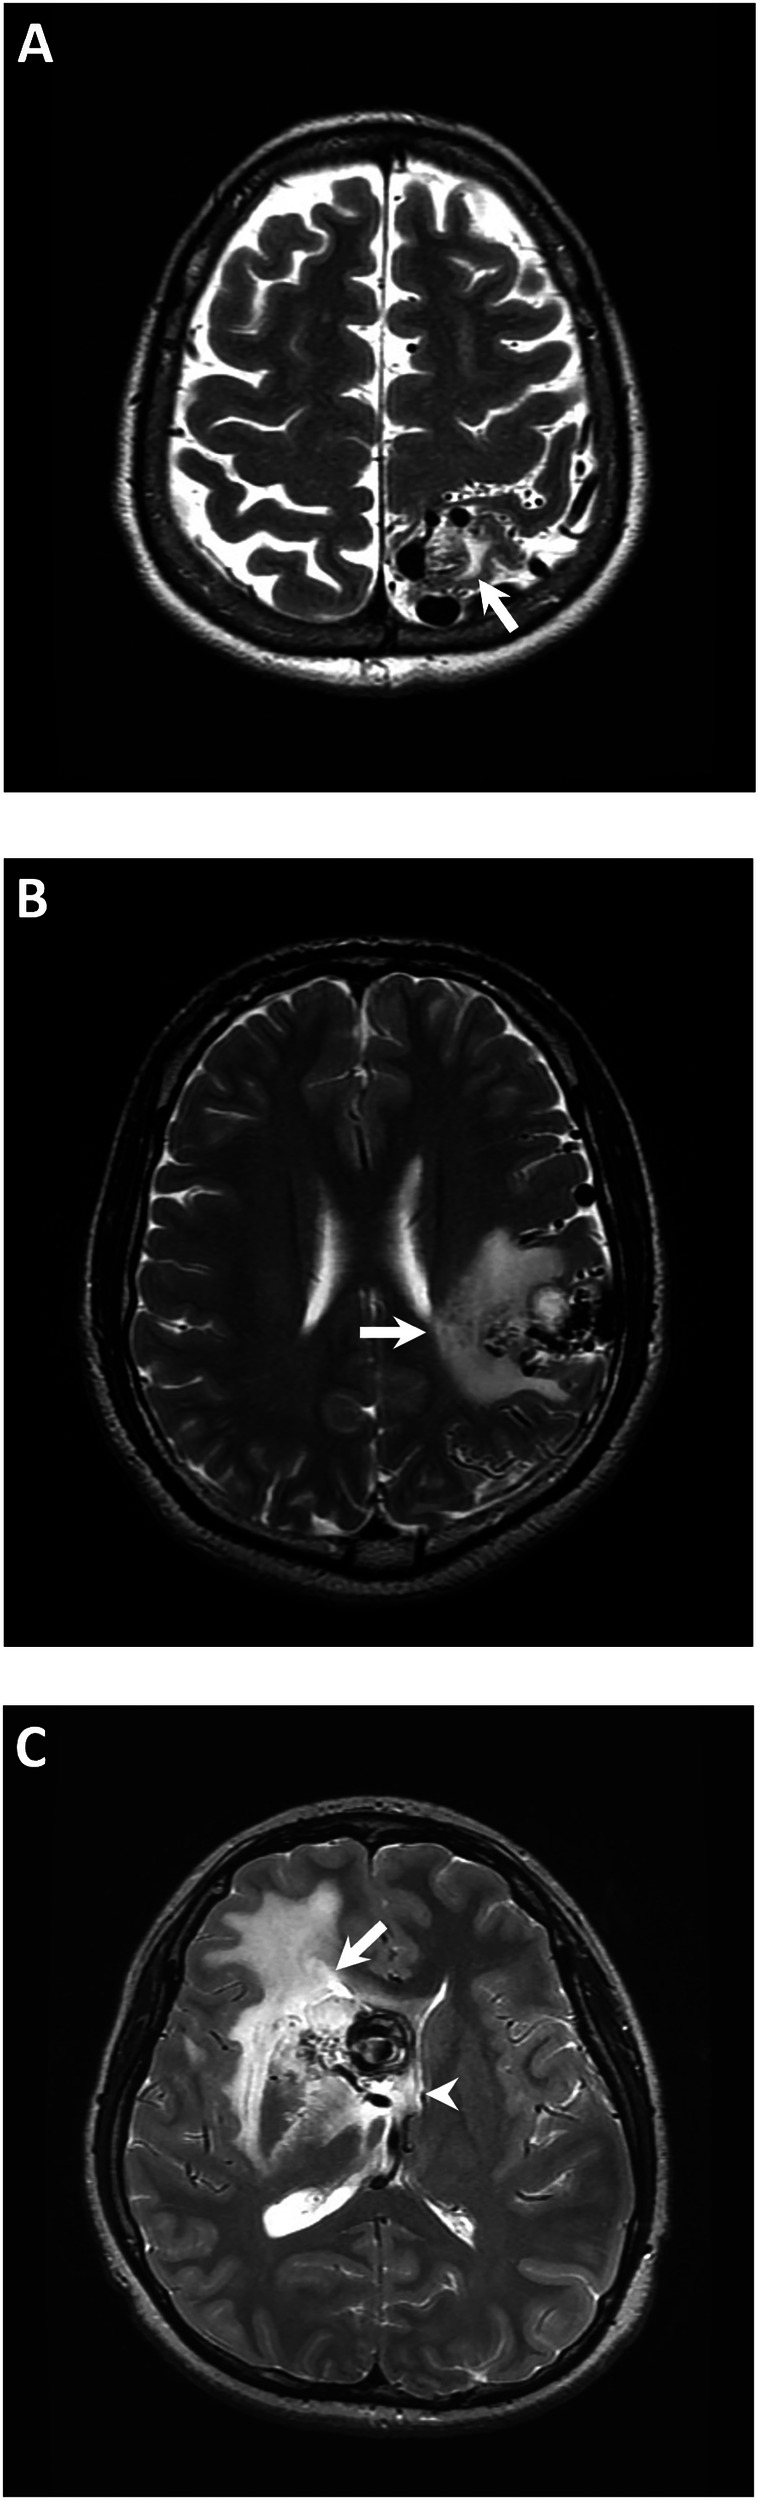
Examples of the three grades of radiation-induced changes (RICs) severity on axial T2-weighted images. A Grade I (mild) RICs at left parietal lobe with increased T2 hyperintensity < 1 cm (arrow) and no mass effect. B Grade II (moderate) RICs at left posterior frontal lobe with increased T2 hyperintensity ≥ 1 cm and mild ventricular compression (arrow). C Grade III (severe) RICs at right frontal lobe with severe mass effect, marked ventricular compression (arrow), and cerebral midline shift (arrowhead)
